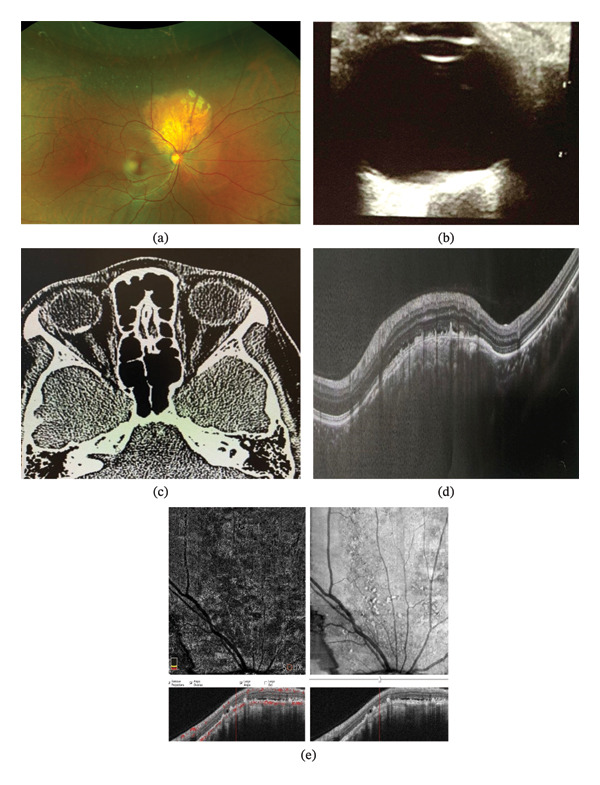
Clinical Case 1: (a) fundus photography—white‐yellowish chorioretinal lesion with patchy pigment deposits, (b) B‐scan ultrasound of the right eye—a hyperechogenic prominent lesion in the posterior pole of the eye globe, (c) CT scan of the right orbit—a plaque‐like intraocular lesion with bone‐equivalent density in the choroid, (d) OCT of the right eye—retinal prominence above the optic nerve head due to a hyperreflective lesion, (e) OCT angiography—subretinal lesion with multiple hyperreflective foci (calcifications) within different retinal layers and shallow neuroepithelial detachment without choroidal neovascularization.
